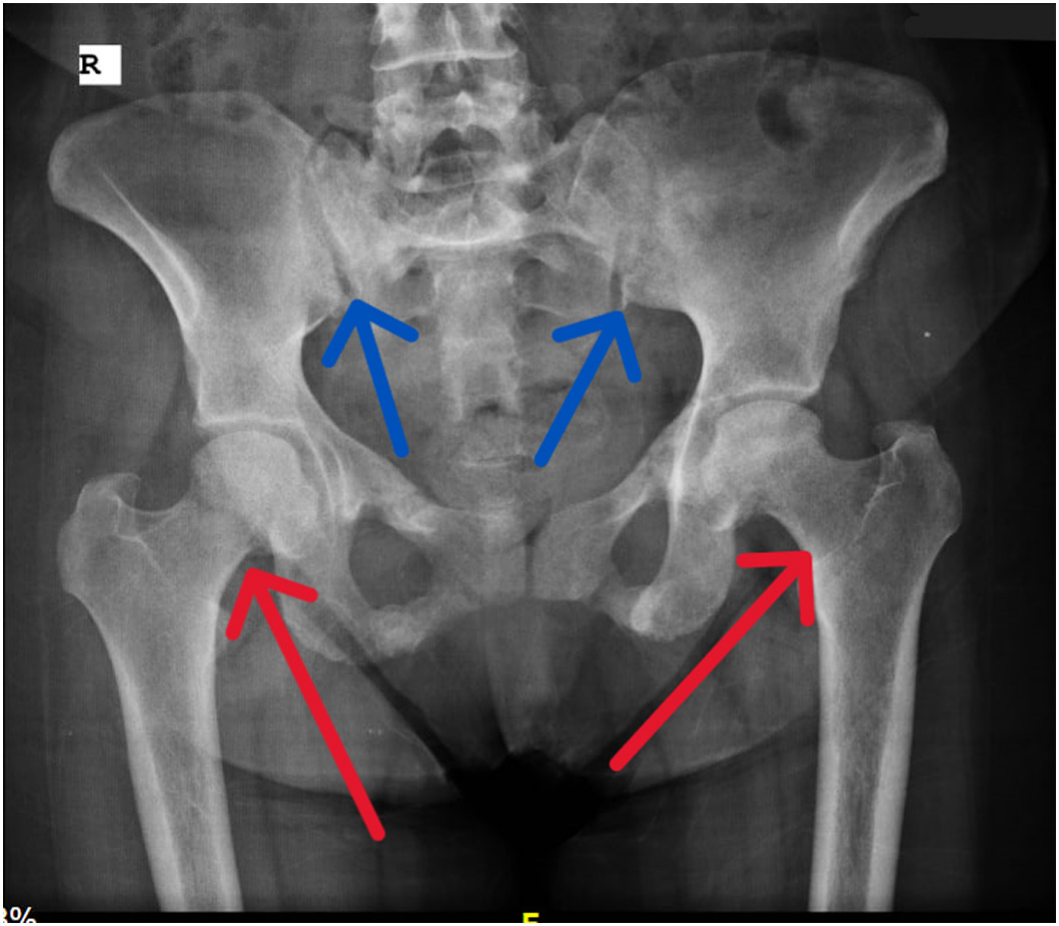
A radiograph pelvic plain X‐ray showed radiolucency in the bilateral femoral neck (Red arrows) and pelvic (blue arrows), suspicious of low bone mineral density (osteoporosis or osteopenia).
